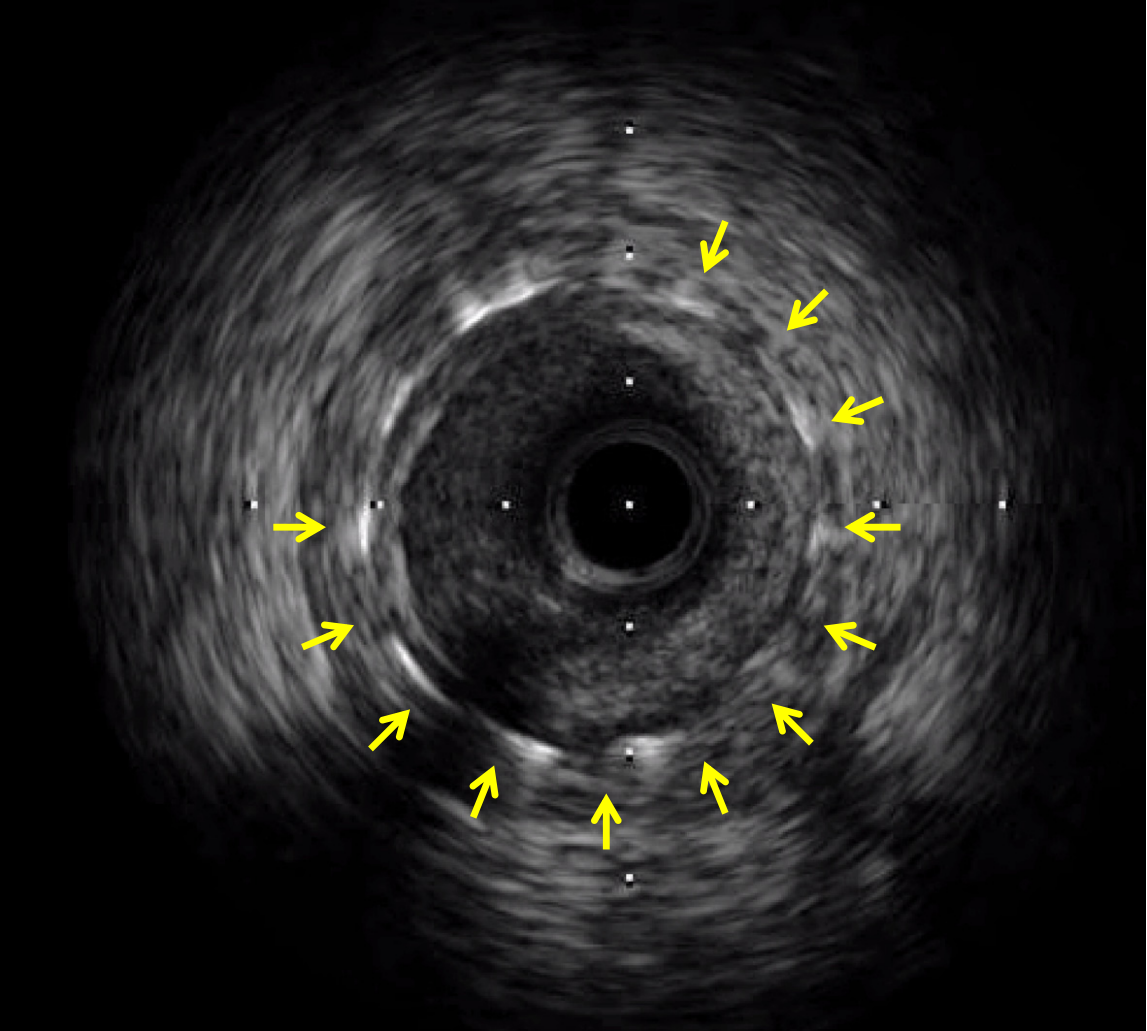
Intravascular ultrasound. Fibrous thrombi are visible in the in-stent segment of the pLAD artery
